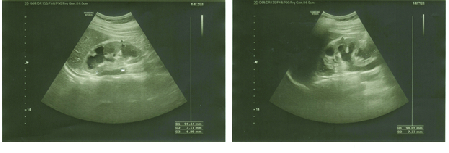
Abdominopelvic sonography in case V.3 showing severe hydronephrosis in both kidneys.
